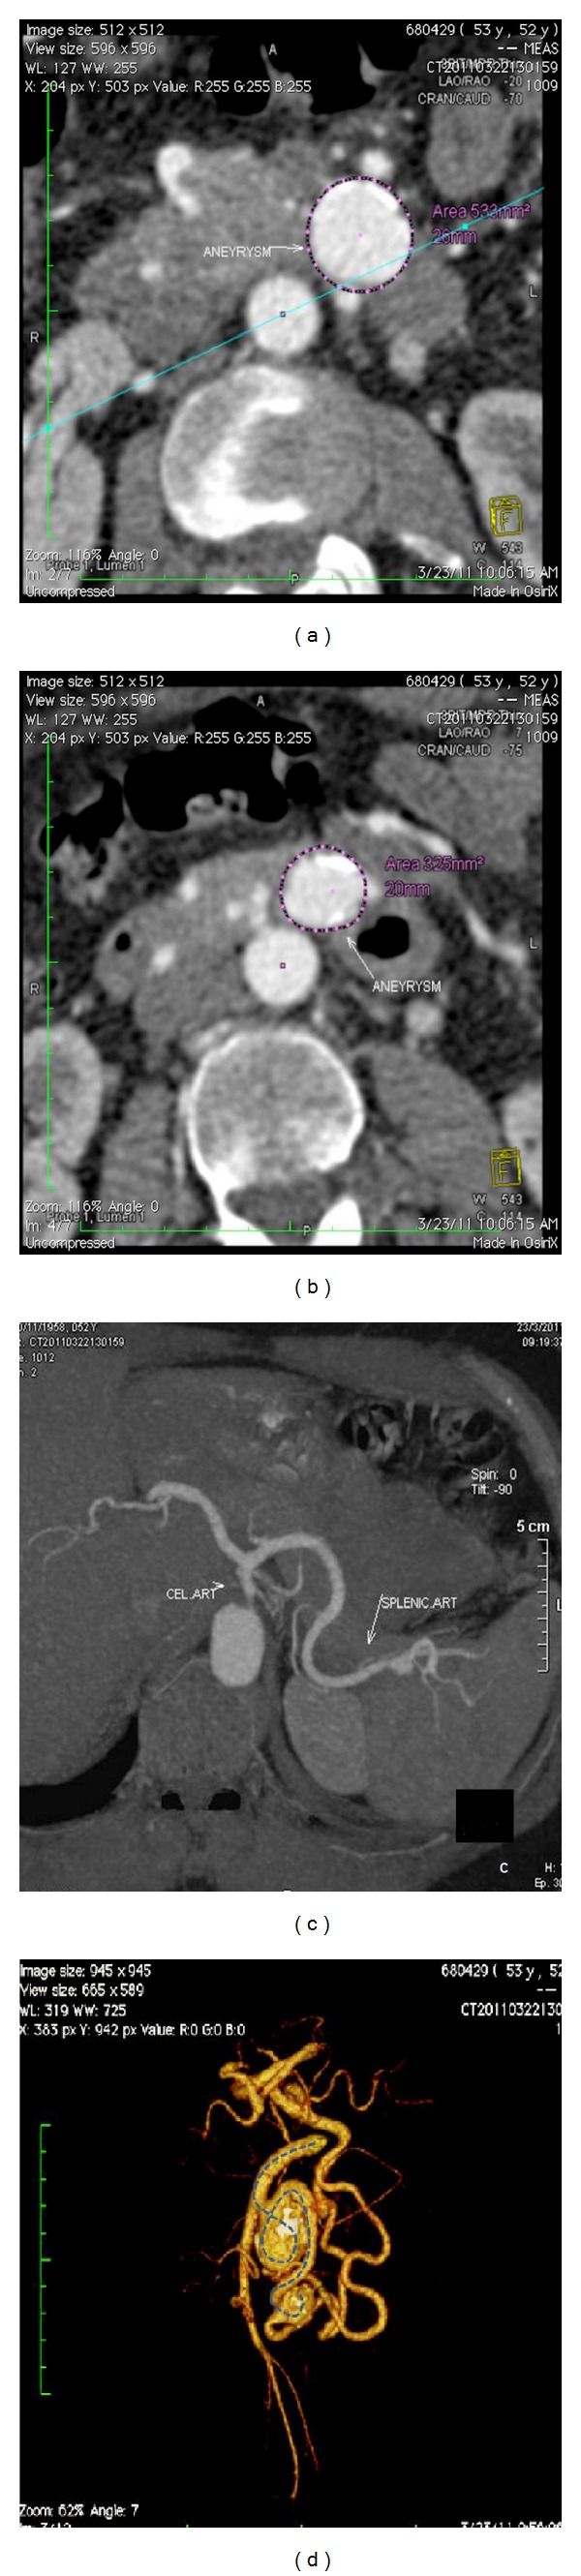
(a, b) Intrapancreatic aneurysms of the IPDA on CTA. (c) Median arcuate ligament-syndrome-like stenosis of the celiac trunk origin. (d) 3D volume rendering image processing provides closeup of the superior mesenteric artery, both IPDA aneurysms and their connecting branch.
